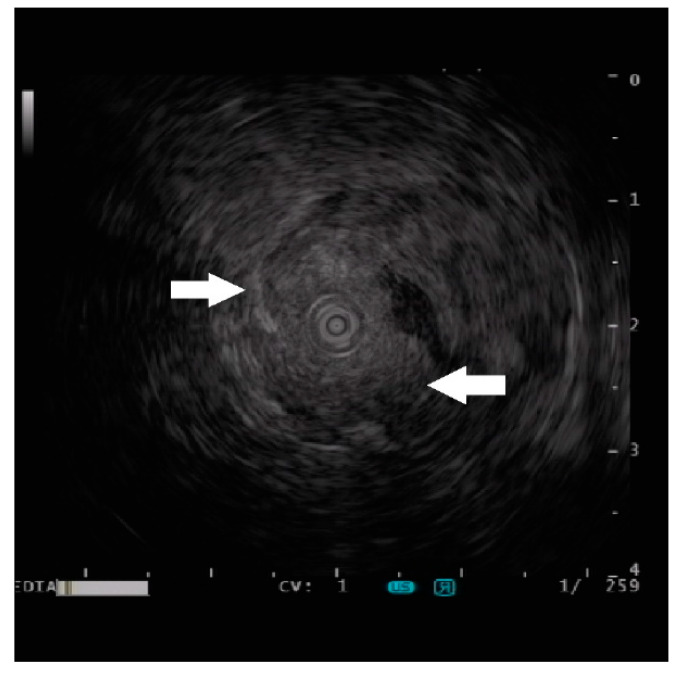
Endoscopic ultrasound image of the lesion (arrows).
